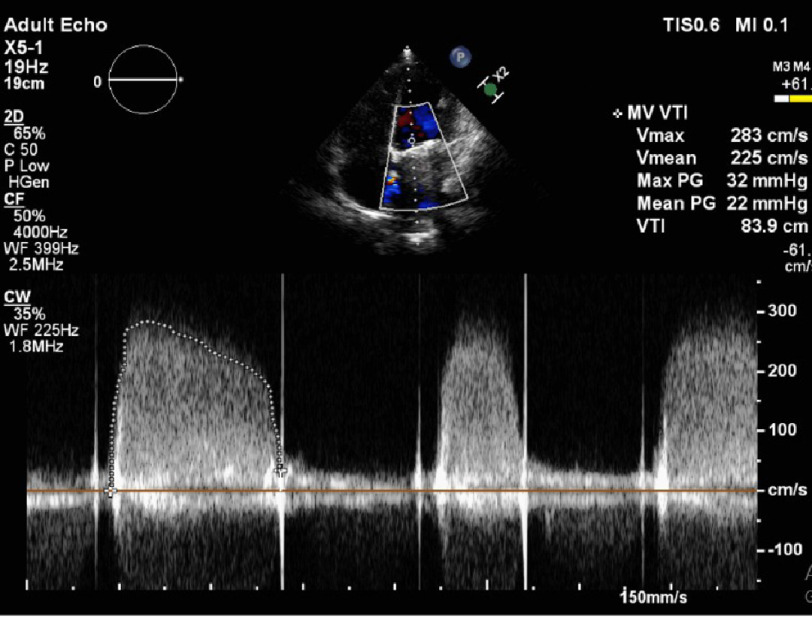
Continuous wave Doppler showing increased gradients across MV (pre-operative).
